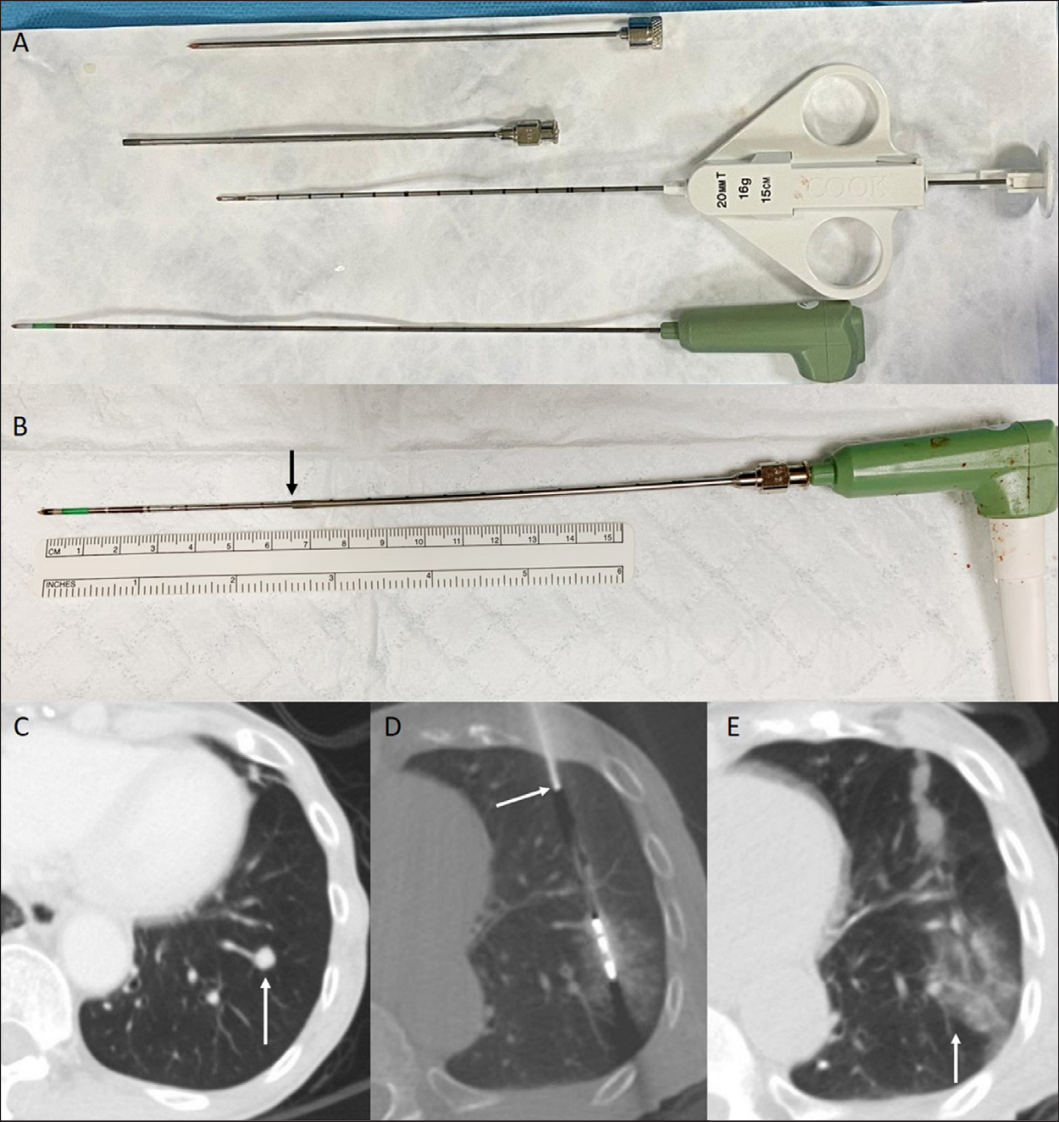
(A) Top to bottom: inner stylet of the guiding needle, 14G/11.6 cm guiding cannula, 16G biopsy needle and 17G/20 cm MWA antenna. (B) A MWA antenna inserted through guiding cannula. Tip of the guiding cannula is indicated by the arrow. (C) CT showed a 0.8 cm metastatic nodule in the left lower lobe. (D) Microwave ablation (MWA) was performed by MWA antenna inserted through the 14G guiding cannula (arrow). (E) Post procedure CT demonstrated satisfactory penumbra covering the nodule (arrow).
